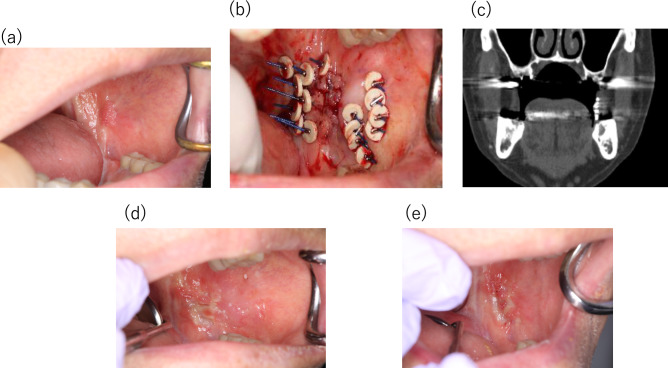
Clinical course of Patient No. 8 with papillary SCC of buccal mucosa after radiotherapy. a A tumor before treatment with size of 12 × 8 × 4 mm. b State of 11 DaRT seeds implanted in a single plane. c Coronal reconstruction CT image of inserted DaRT seeds; d Mucositis appeared 1 weeks after seed removal. e Mucosal erosion 12 weeks after treatment. Biopsy showed no malignancy
